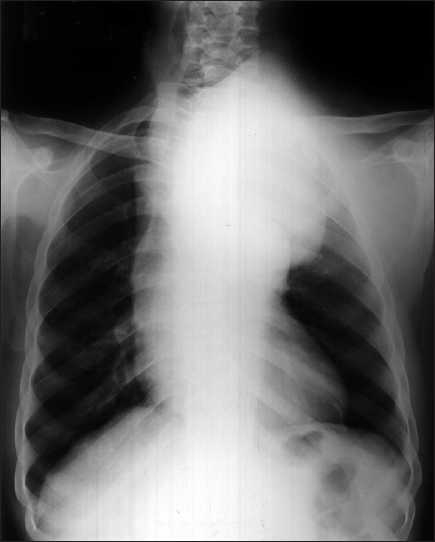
Chest roentgenogram showing widening of the mediastinum with a radiopaque mass extending into the superior mediastinum occupying the left upper and mid-zone with a right tracheal shift
